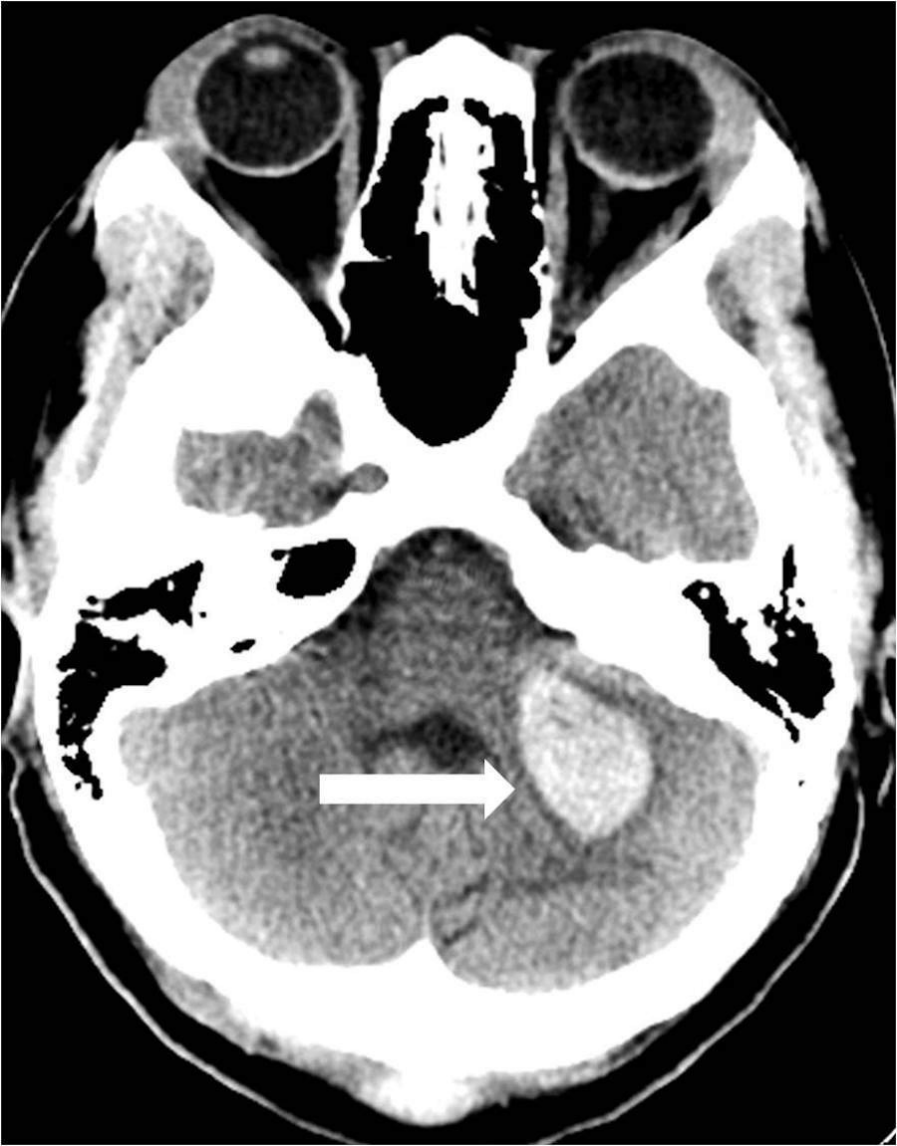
Initial head computed tomography reveals an acute intraparenchymal hemorrhage in the left cerebellar hemisphere (arrow).
